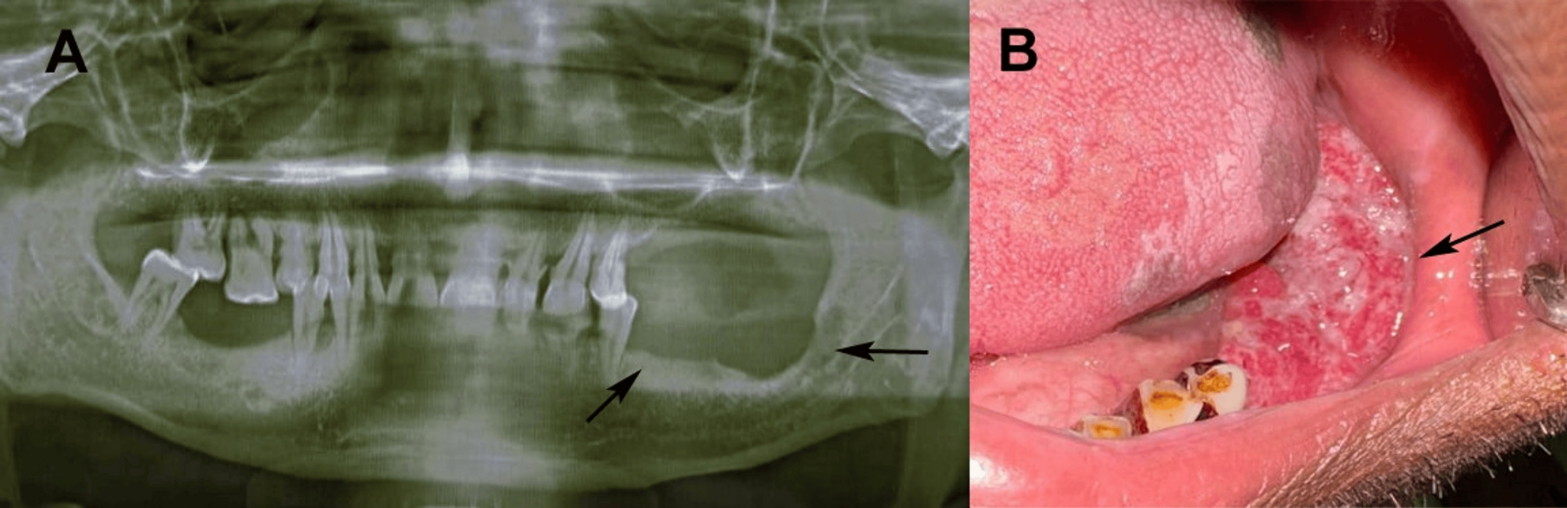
(A) Panoramic radiograph (OPG) showing alveolar ridge erosion from the premolar to the third molar area (black arrow) and (B) intraoral examination showing non-homogeneous irregular exophytic growth extended from the lower left second premolar area to third molar area. Original patient's images, used with patient's consent for publication.
